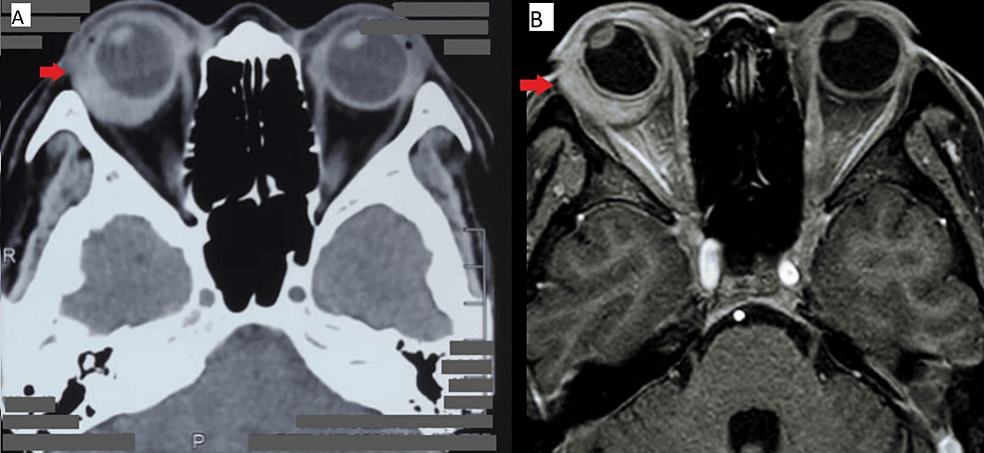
Contrasted computed tomographic scan and magnetic resonance imaging of the brain and orbit demonstrating enhancing hyperdense lesion at right lateral wall measuring 2.0 x 0.5 x 1.8 cm, highlighted with red arrows. 5A: Contrasted computed tomographic scan of the brain and orbit 5B: Magnetic resonance imaging of the brain and orbit
